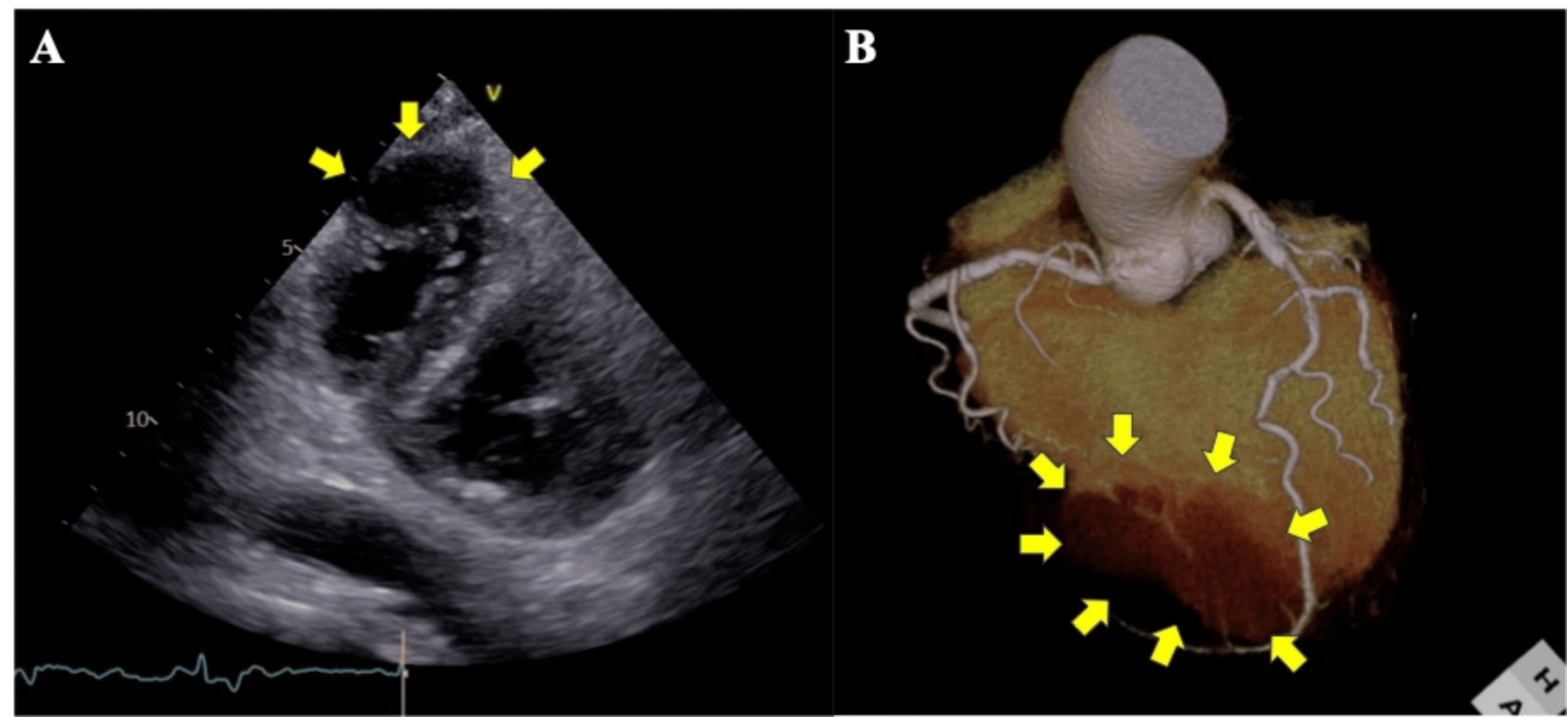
Preoperative transthoracic echocardiography (A) and enhanced three-dimensional computed tomography (B) images. A: Left ventricular short-axis view showing a tumor shadow continuous with the right ventricle (arrow). B: Left anterior descending branch with 75% stenosis and a pseudo-false aneurysm (arrow).
